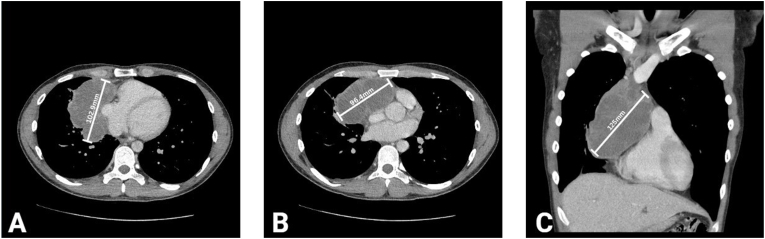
CT chest with IV contrast demonstrating a large anterior mediastinal mass with concomitant superior mediastinal lymphadenopathy. (A) Axial cut of the mediastinal mass with a largest anteroposterior diameter of 102.9mm. (B) Axial cut of the mediastinal mass with largest transverse diameter of 96.4mm. (C) Coronal cut with a peak diameter of 125mm.
